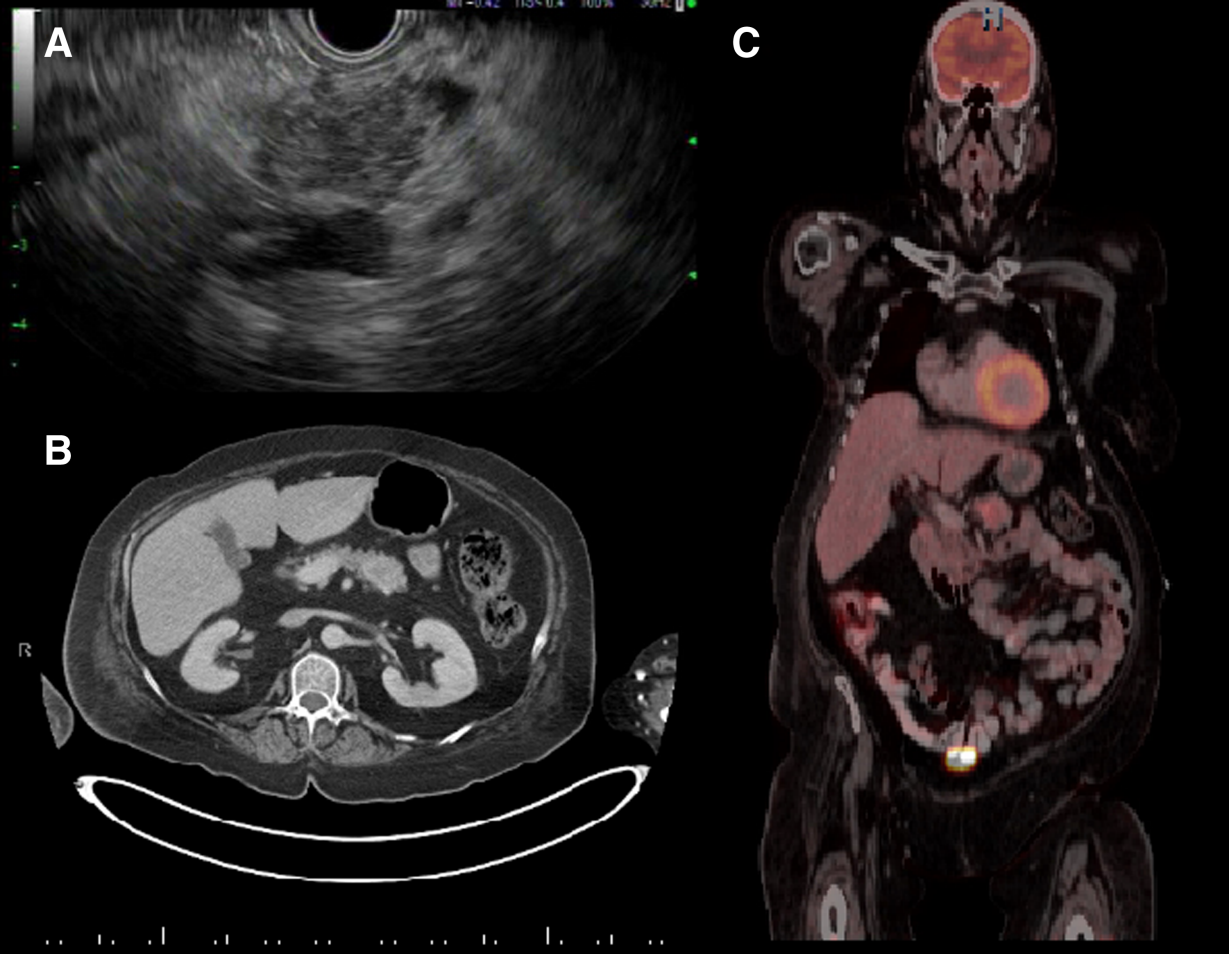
(A) Endoscopic ultrasound of pancreatic mass. (B) CT abdomen of the pancreatic mass, also demonstrating a concerning perihepatic lymph node that was proven positive on final pathology. (C) PET-CT demonstrating mild PET avidity of the pancreatic mass. CT, computed tomography; PET-CT, positron emission technology-CT.
